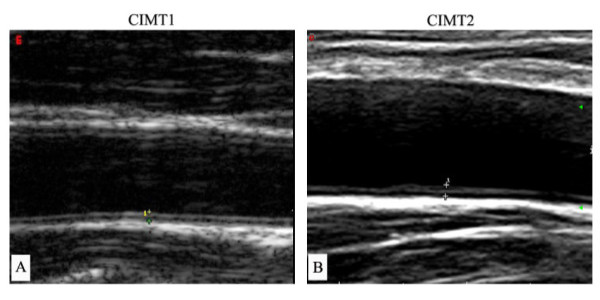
B-mode tomographic ultrasound figures at CIMT1 and CIMT2. The distance between two cross marks: carotid intima-media thickness (CIMT). (A: using Esaote DU3, Italy in 2006; B: using Esaote Mylab90, Italy in 2010).
